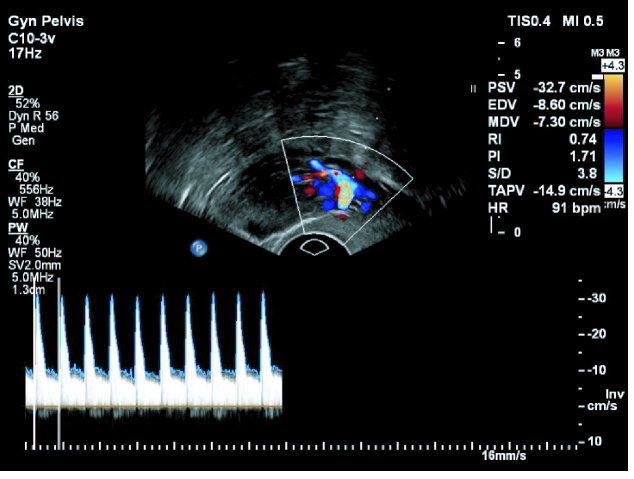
Uterine artery Doppler, by color Doppler ultrasound.
